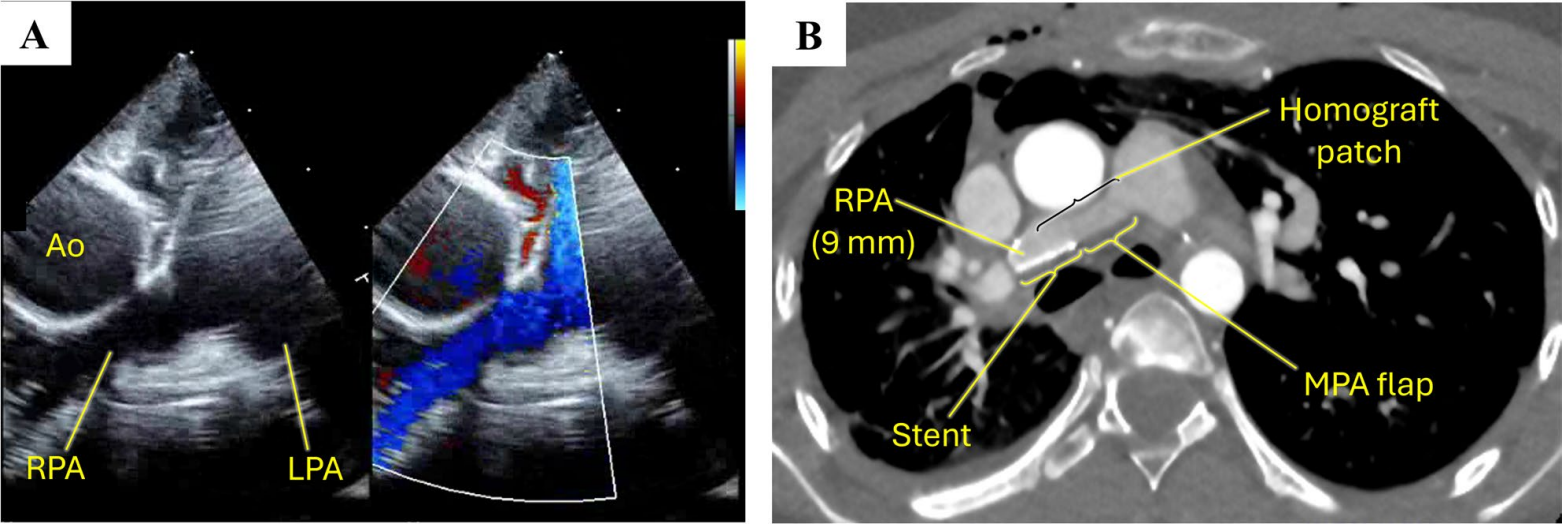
A: Postoperative echocardiography for RPA, B: postoperative chest computed tomographyA: Postoperative echocardiography for RPA, B: postoperative chest computed tomography Ao = aorta, LPA = left pulmonary artery, MPA = main pulmonary artery, RPA = right pulmonary artery
